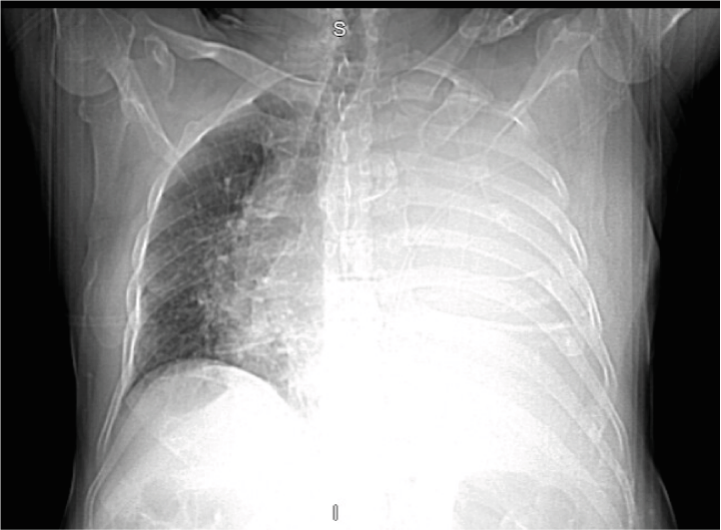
Chest radiograph showing total opacification of the left hemithorax with a shift of the mediastinum to the right side.
